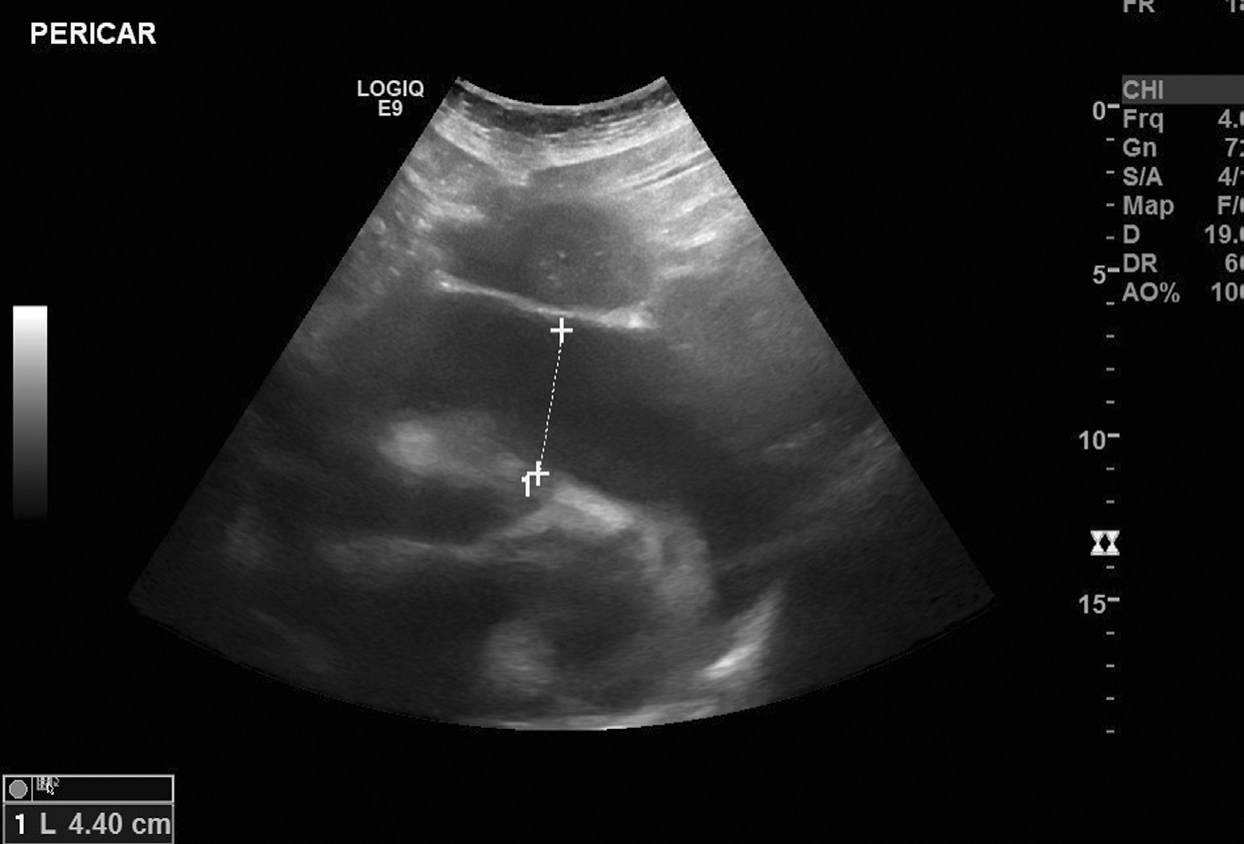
Transthoracic echocardiogram (TTE) revealing a large circumferential pericardial effusion creating an echo-free space of 4,6 cm and a swinging heart.
